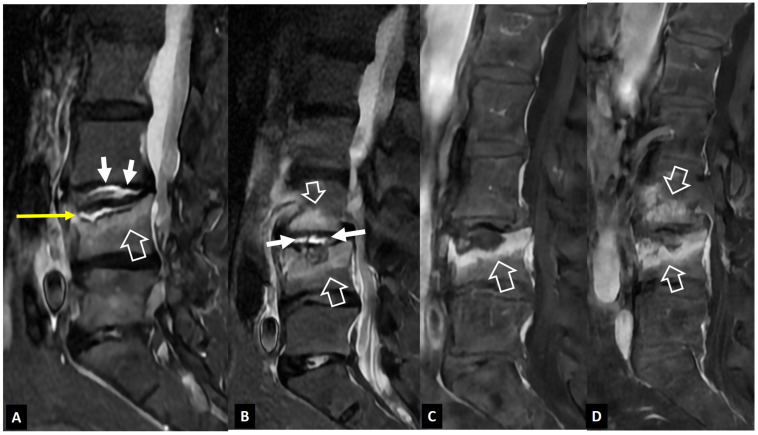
A 67-year-old female with low back pain for 3 weeks, following a minor trauma. A recent DEXA showed osteoporosis. Sagittal (A) and left parasagittal (B) STIR MR images, and corresponding fat-suppressed contrast-enhanced T1-w MR images (C,D), showing bone marrow edema on both sides of the L3–L4 disc space with enhancement (open arrows). A “fluid” sign (yellow arrow in (A)) and a linear delamination injury of the disc (arrows in (A,B)) do not show enhancement after contrast administration.
